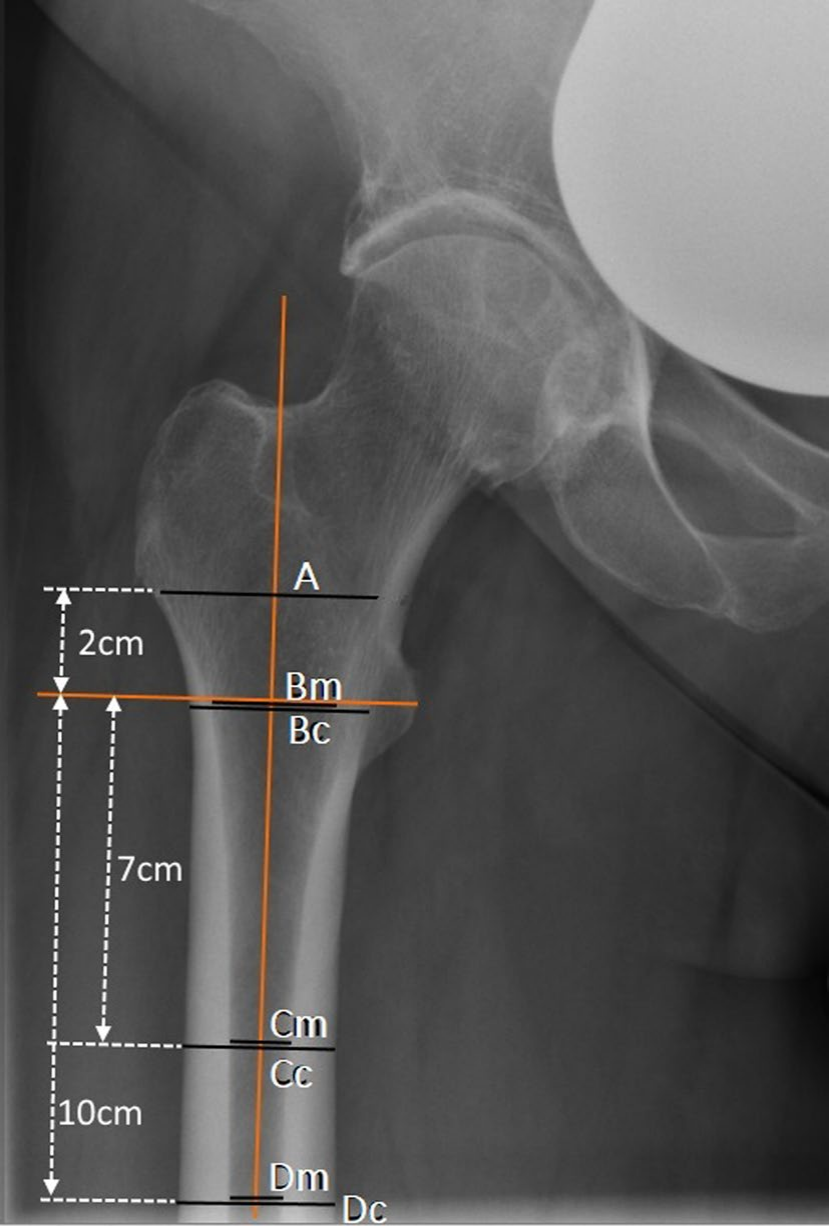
Measurement of the indices on plain ap radiographs: canal-bone-ratio CBR-7 cm (= Cm/Cc) and CBR-10 cm (= Dm/Dc), canal-calcar ratio CCR (= Dm/Bm); canal flare index CFI (= A/Dm).
